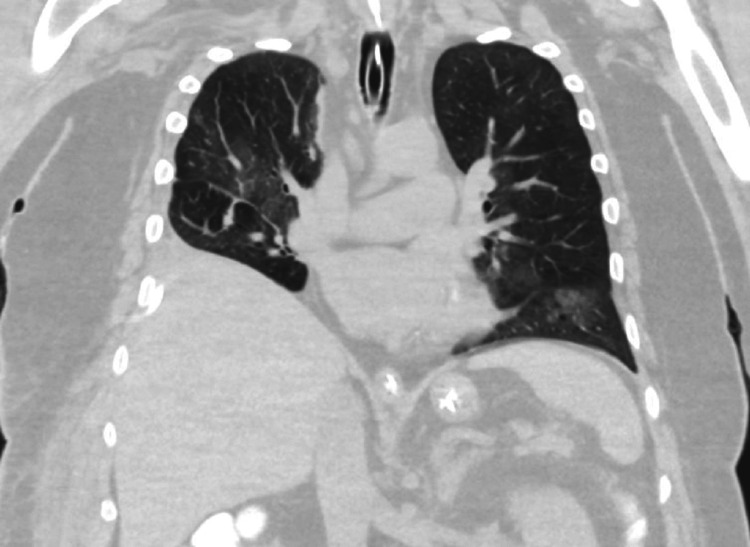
CT chest revealing resolution of the pneumonia
